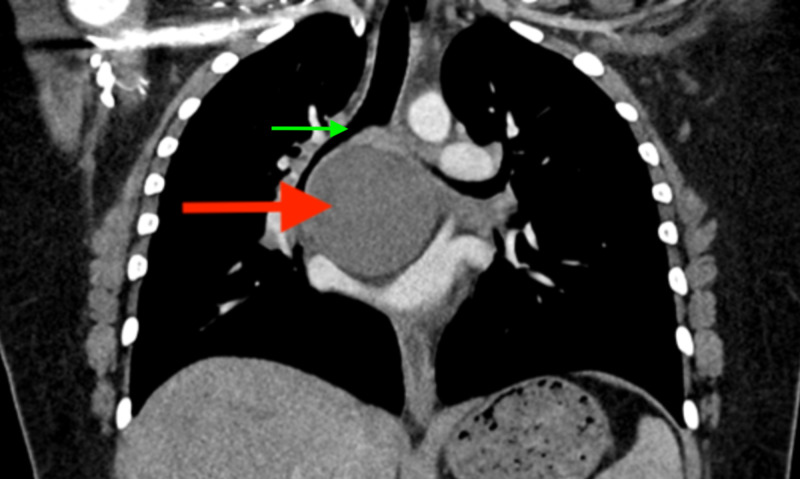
Chest CT (coronal view) with intravenous contrast showing thin-walled cyst (red arrow) in the subcarinal region with internal minimally complex fluid density. The cyst splays the right and left mainstem bronchus (green arrow).
